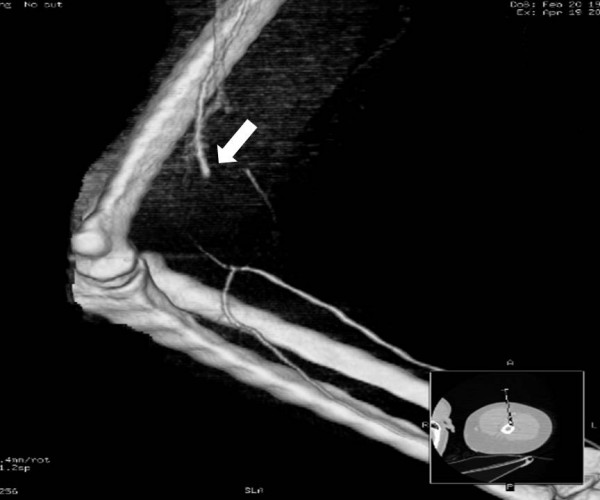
Same case as in figures 3, 4. CT-angiography with 3D-reconstruction demonstrating a 4 to 5 cm long brachial artery laceration at the elbow (arrow) with reconstitution of forearm vessel flow via collateral perfusion.
